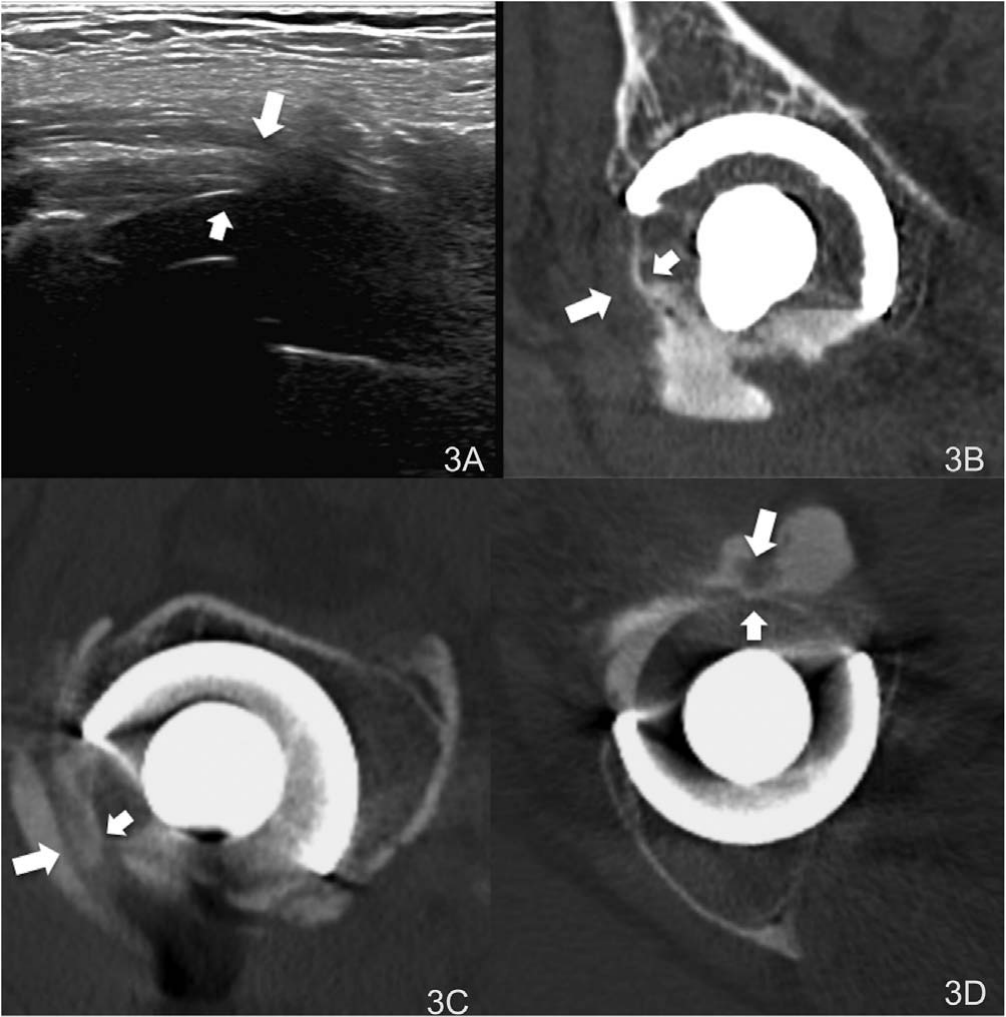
(A) Ultrasonography showing the DM liner (small arrow) and the iliopsoas tendon (big arrow). Arthro-CT-scan: impingement between the DM liner (small arrows and iliopsoas tendon (big arrow) showed in the sagittal (B), coronal oblique (C) and axial plane (D).
